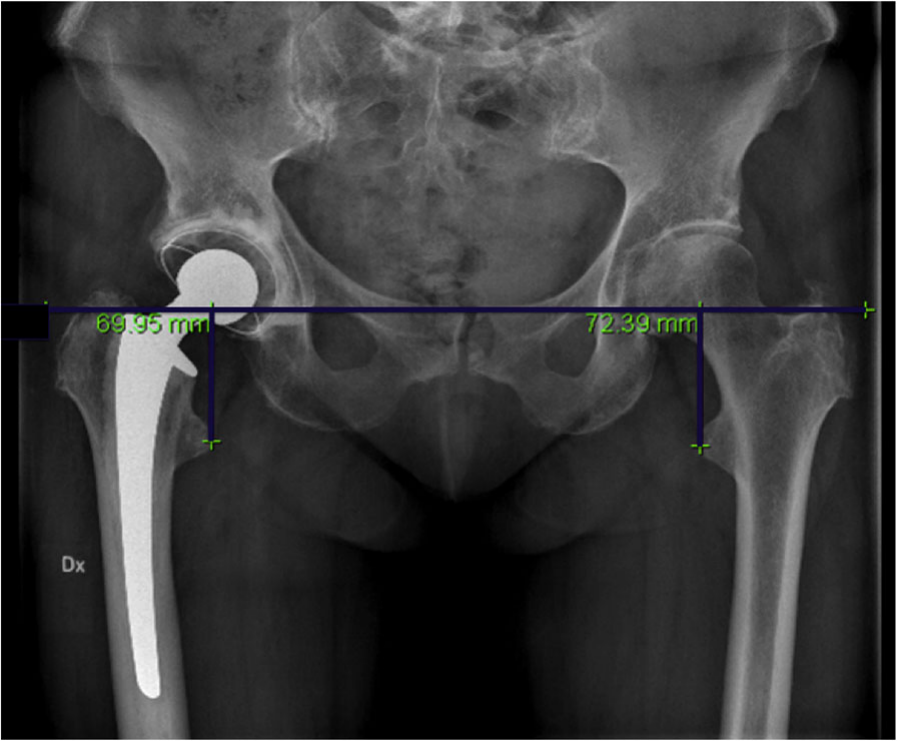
Plain radiograph measurement of the leg length discrepancy as the perpendicular distance between a line passing through the lower edge of the teardrop points to the corresponding tip of the lesser trochanter
